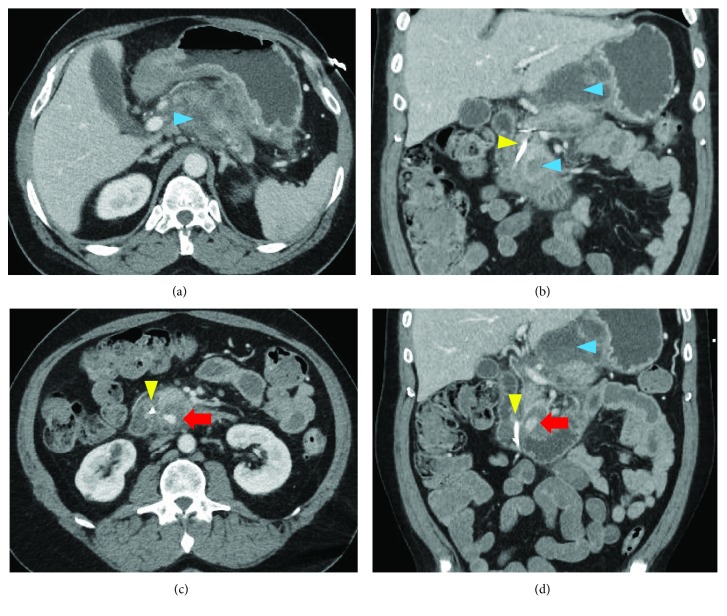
CT showing pancreas with necrotic fluid collections on axial (a) and coronal (b) sections. Contrast extravasation into the IPDA pseudoaneurysm (red arrows) on axial (c) and coronal (d) sections. Yellow arrowheads point to pancreatic duct stent. Blue arrowheads point to pancreatic fluid collections.
